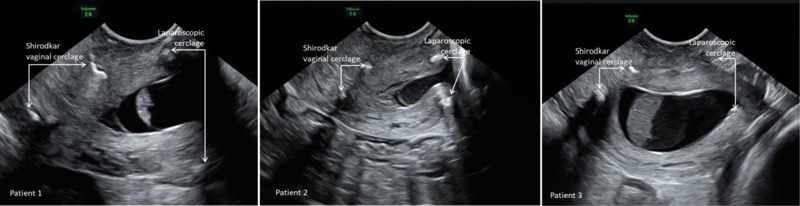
Ultrasonography images of the patients' cervixes after Shirodkar vaginal cerclage.
